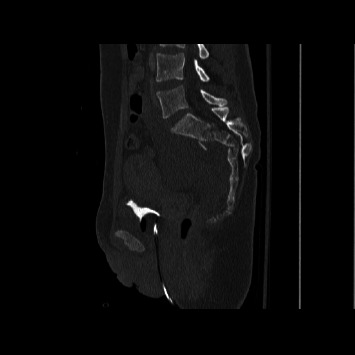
Sagittal CT showing an AOSpine type C3 sacral fracture.
